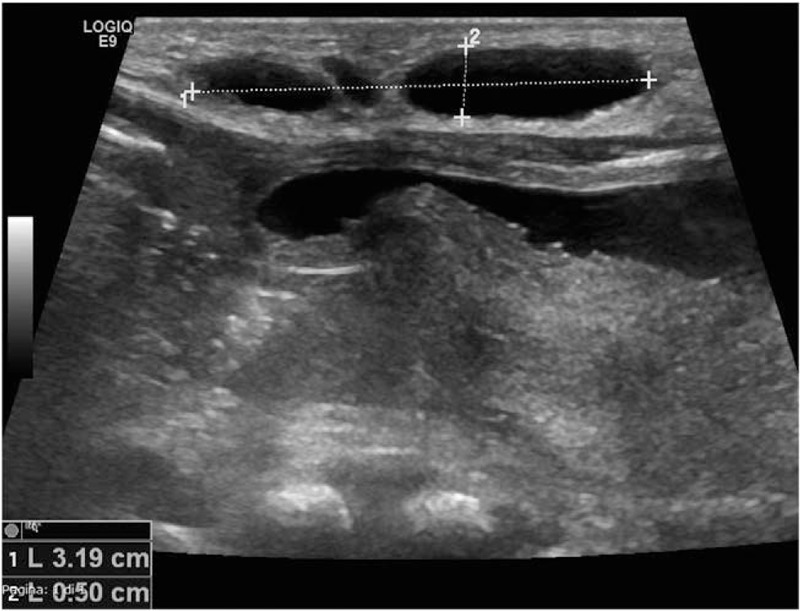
Abdominal ultrasound scan showing subcutaneous cysts. 1: maximum diameter 3.1 cm, 2: minimum diameter 0.5 cm.
